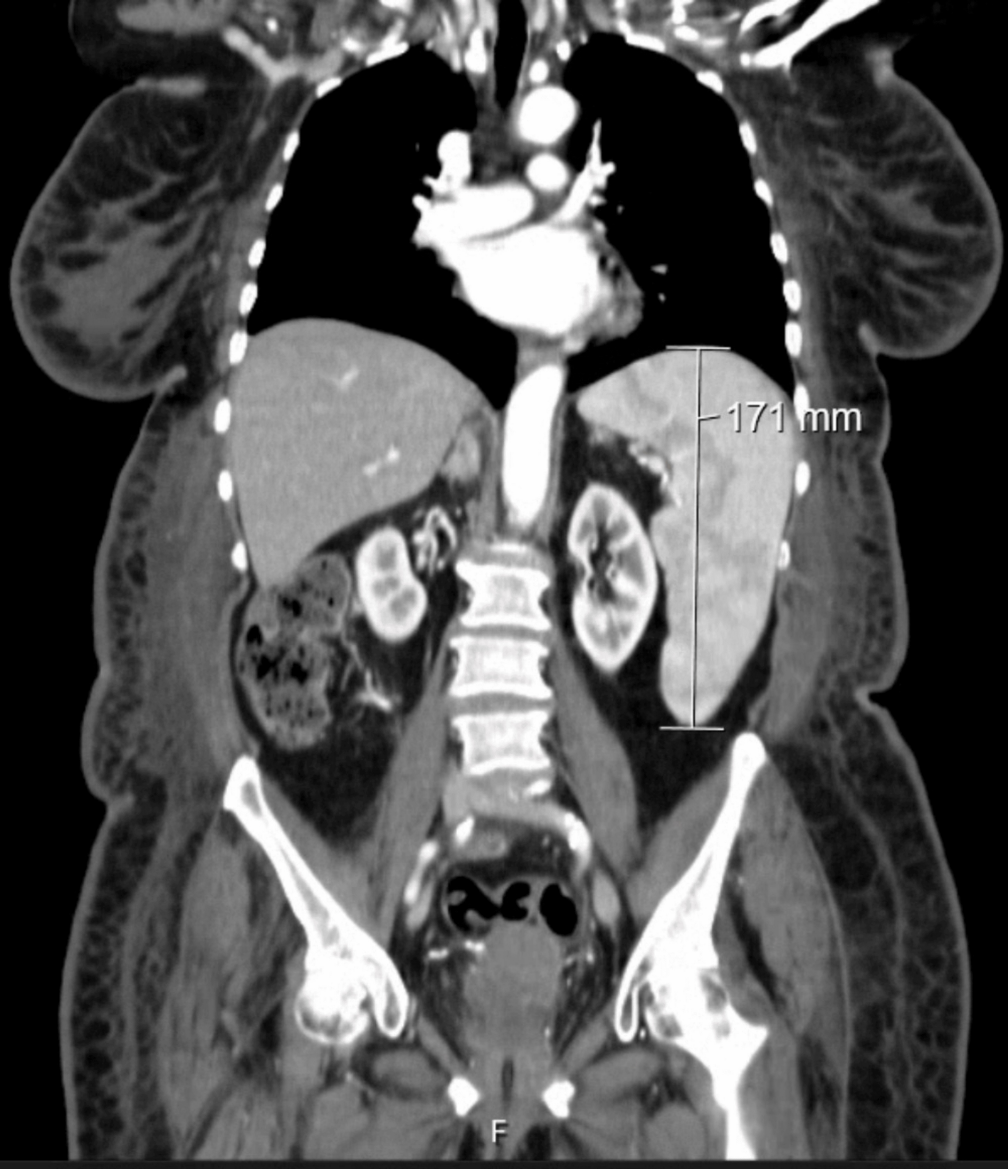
Computed tomography showing an enlarged spleen
